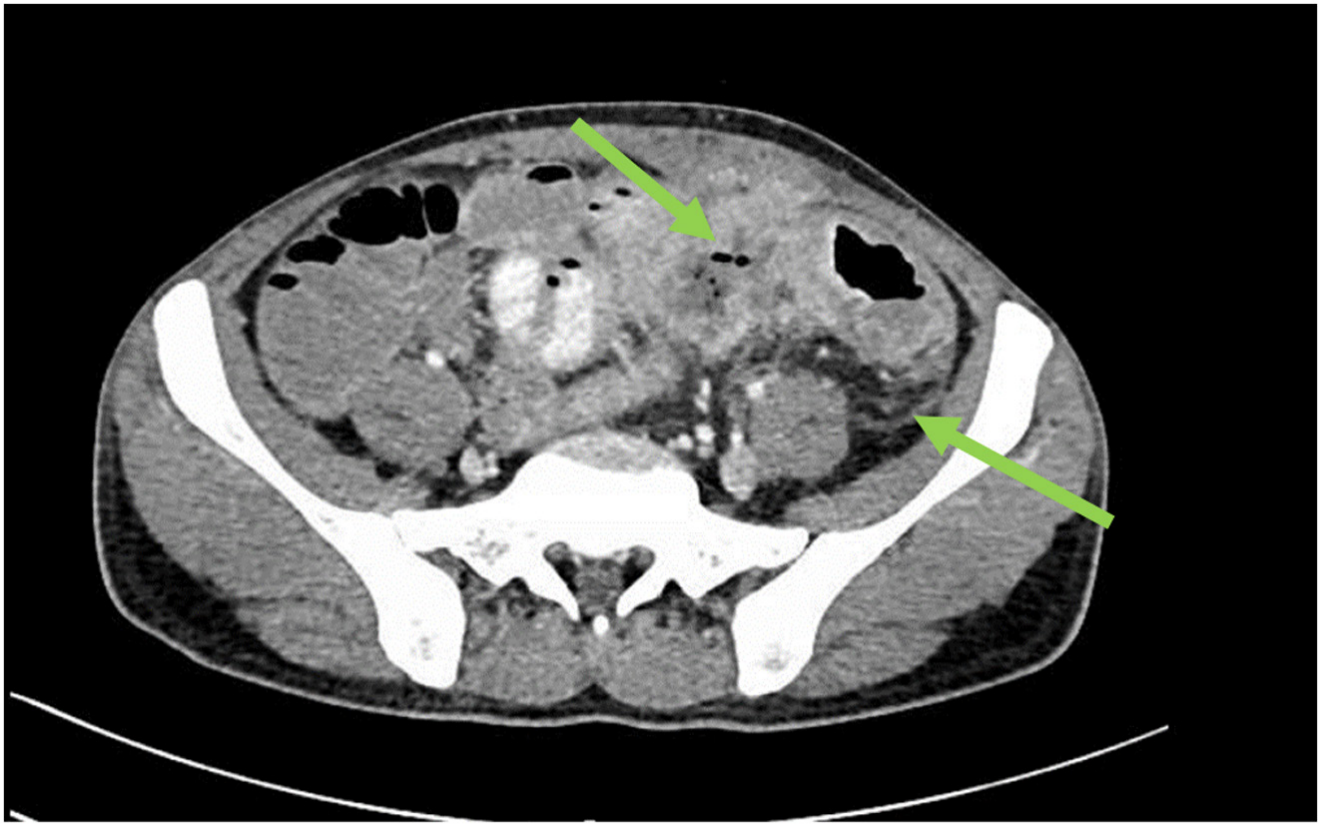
Abdominal CT scan showed thickened sigmoid colon with abdominal lymphadenopathy (arrows).
